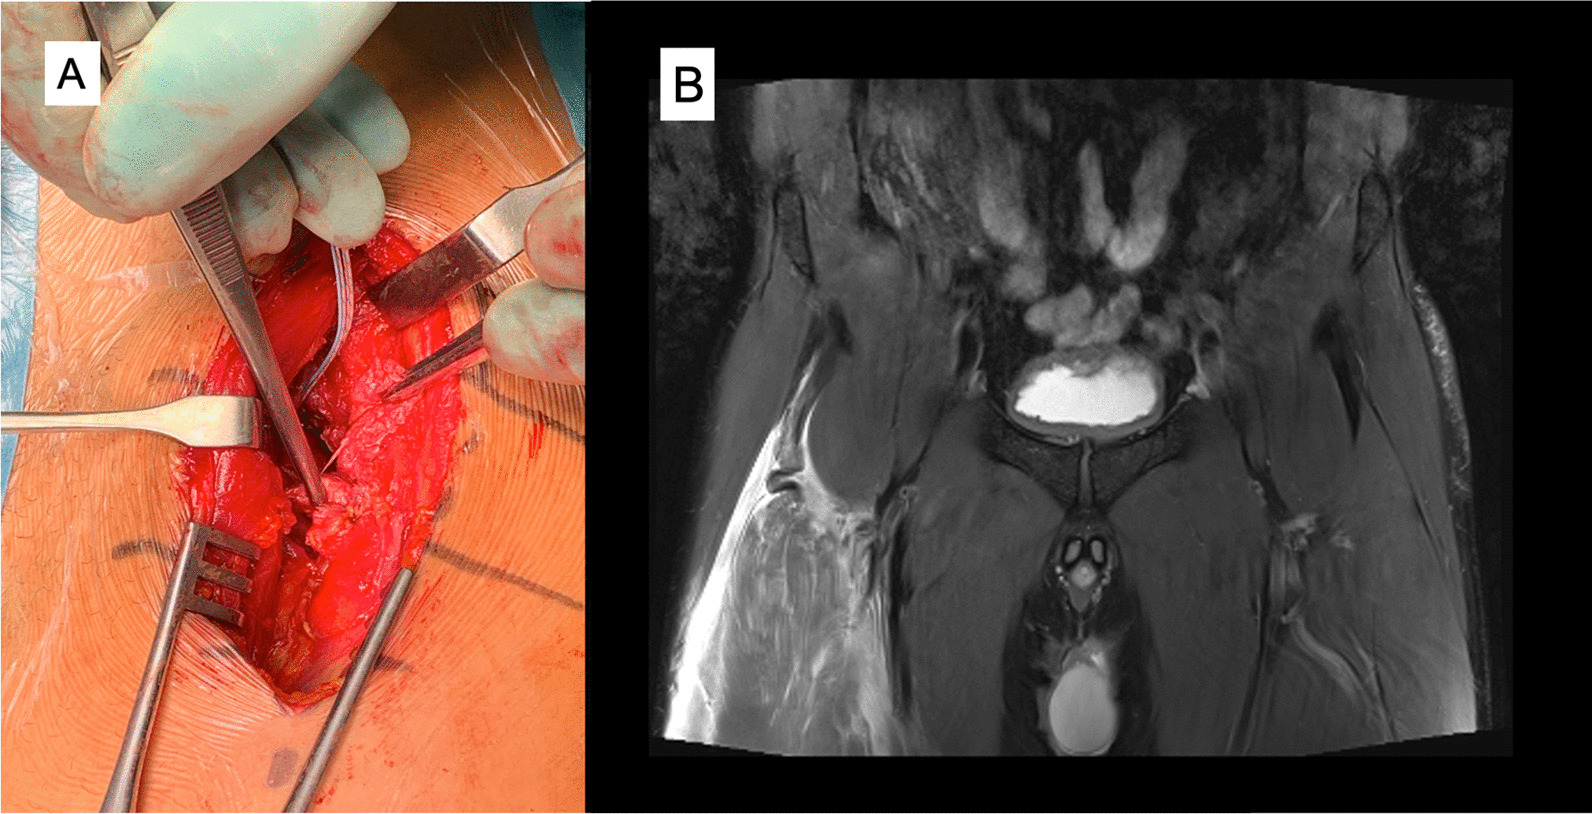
Complete proximal rectus femoris tendon rupture: operative imaging (A) and coronal proton density fat saturated image that shows distal retraction of the muscle and the “waving” pattern of the tendon lost tension MRI (B)
